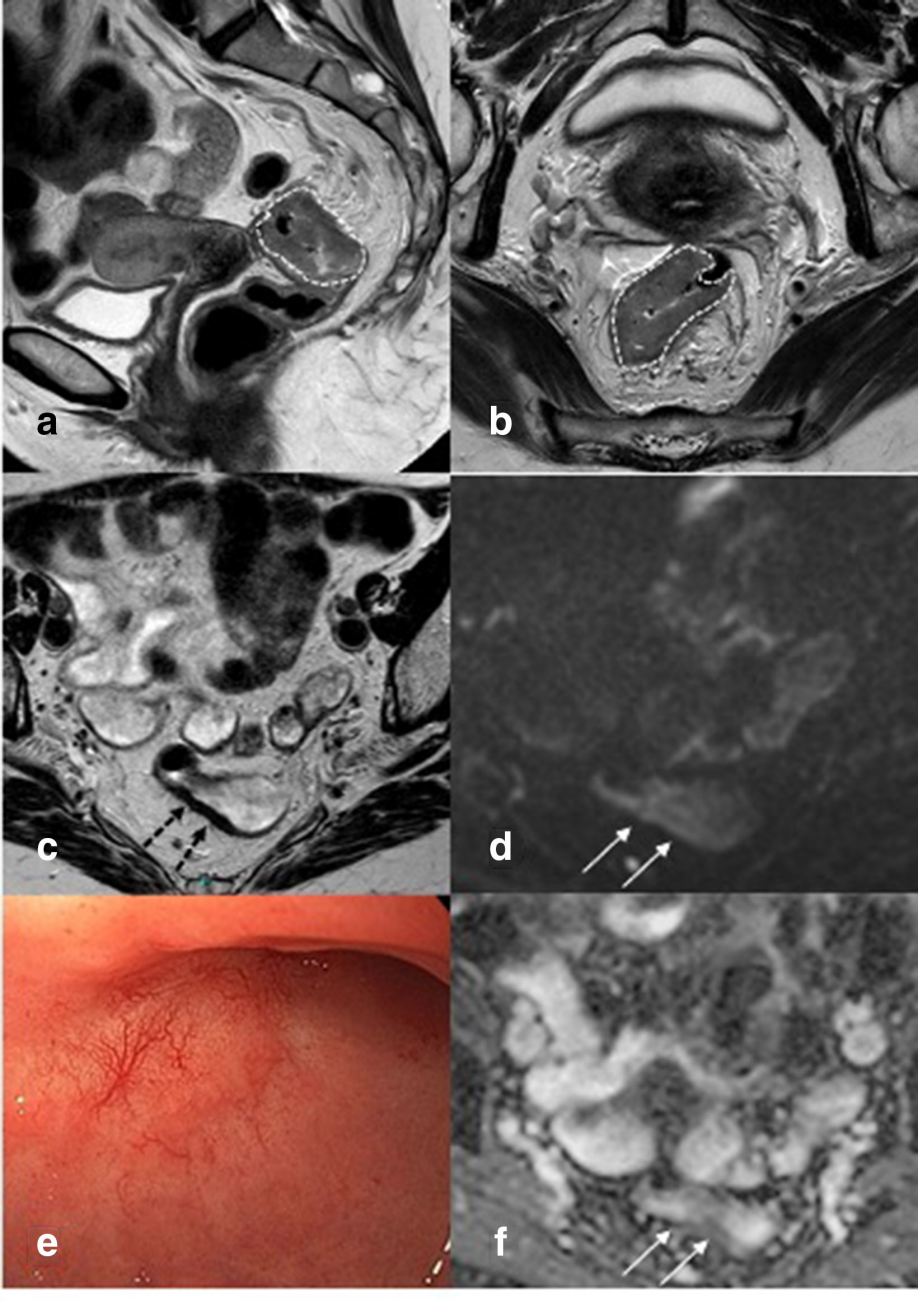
Complete response. Patient with a proximal rectal tumour with extramural growth on outlined with a dotted line on T2-weighted imaging on a and b before treatment. After chemoradiation (c, d, f) on the T2-weighted axial image (c, arrows) homogeneous fibrosis is visible at the former tumor location. At diffusion-weighted imaging, no residual restriction (d, f) is found. Endoscopy shows the typical image of a complete response: a white area with teleangiectasia (e).
